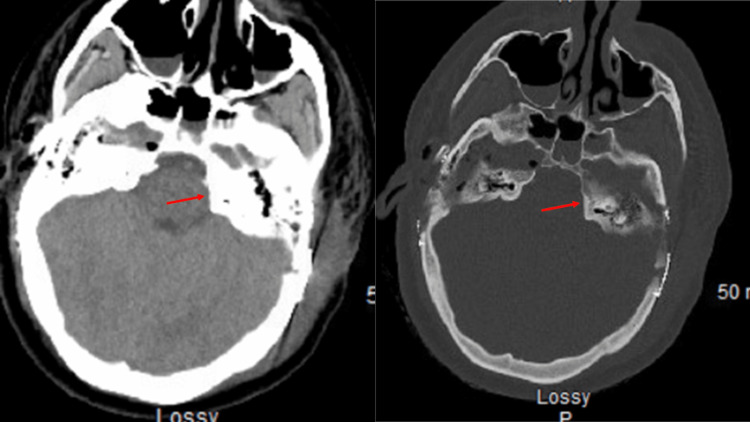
Postoperative axial head CT The view shows no compression on the trigeminal nerve after drilling out the protruding petrous bone CT: computed tomography
